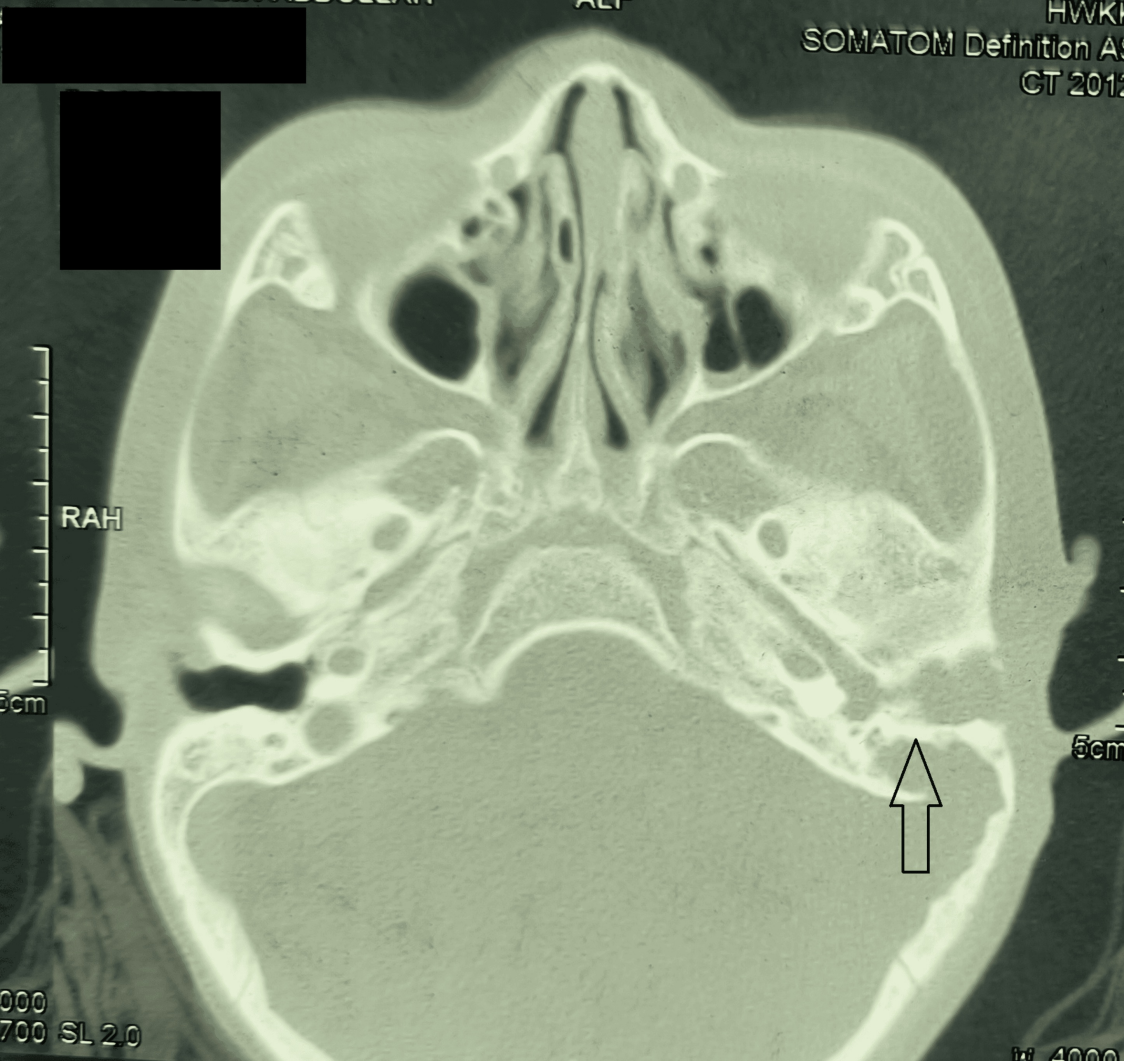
CT in axial section of the temporal bone. It showed a heterogeneous enhancing lesion seen within the left side of the ear canal and mastoid cavity. The ossicles were not visualized, and the scutum was eroded. The lateral semicircular canal appeared to have a dehiscence as well. Posteriorly, there was a rim enhancement at the left cerebellar region measuring 2.2 (anteroposterior) x 1.8 (width) x 1.8 (craniocaudal). No evidence of hydrocephalus was present, but there was a mass effect causing compression to the adjacent sigmoid and transverse sinus; however, it was preserved. The facial nerve canal was intact.
